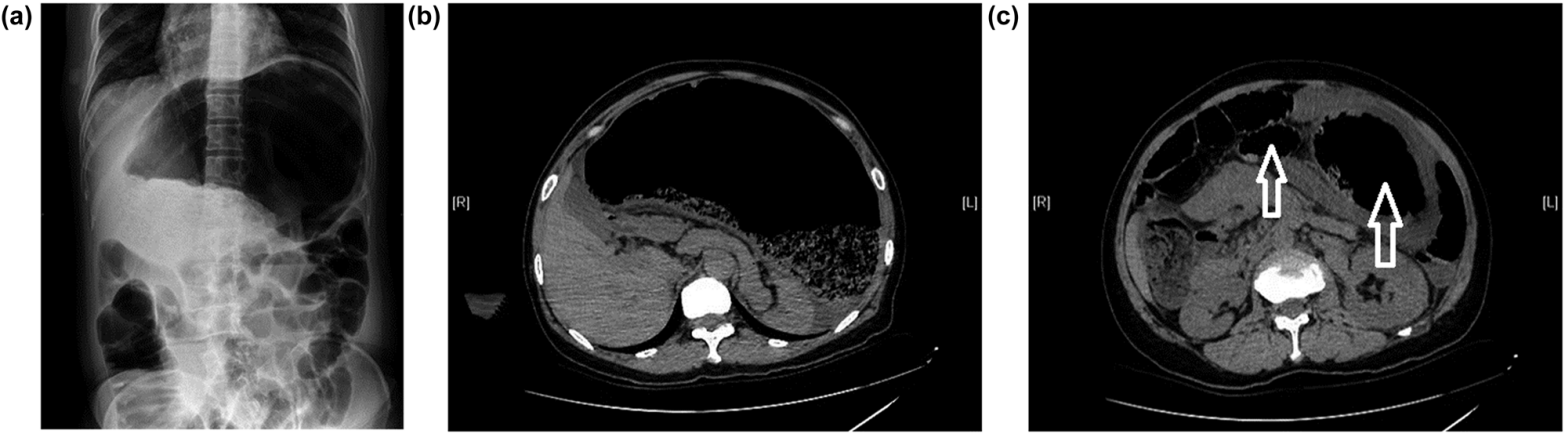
Imaging examination of the transverse colonic duplication. (a) Abdomen film with the patient in the erect position. (b) CT scan shows a giant cyst in the abdominal cavity. (c) The arrows indicate the transverse colon and duplication.
